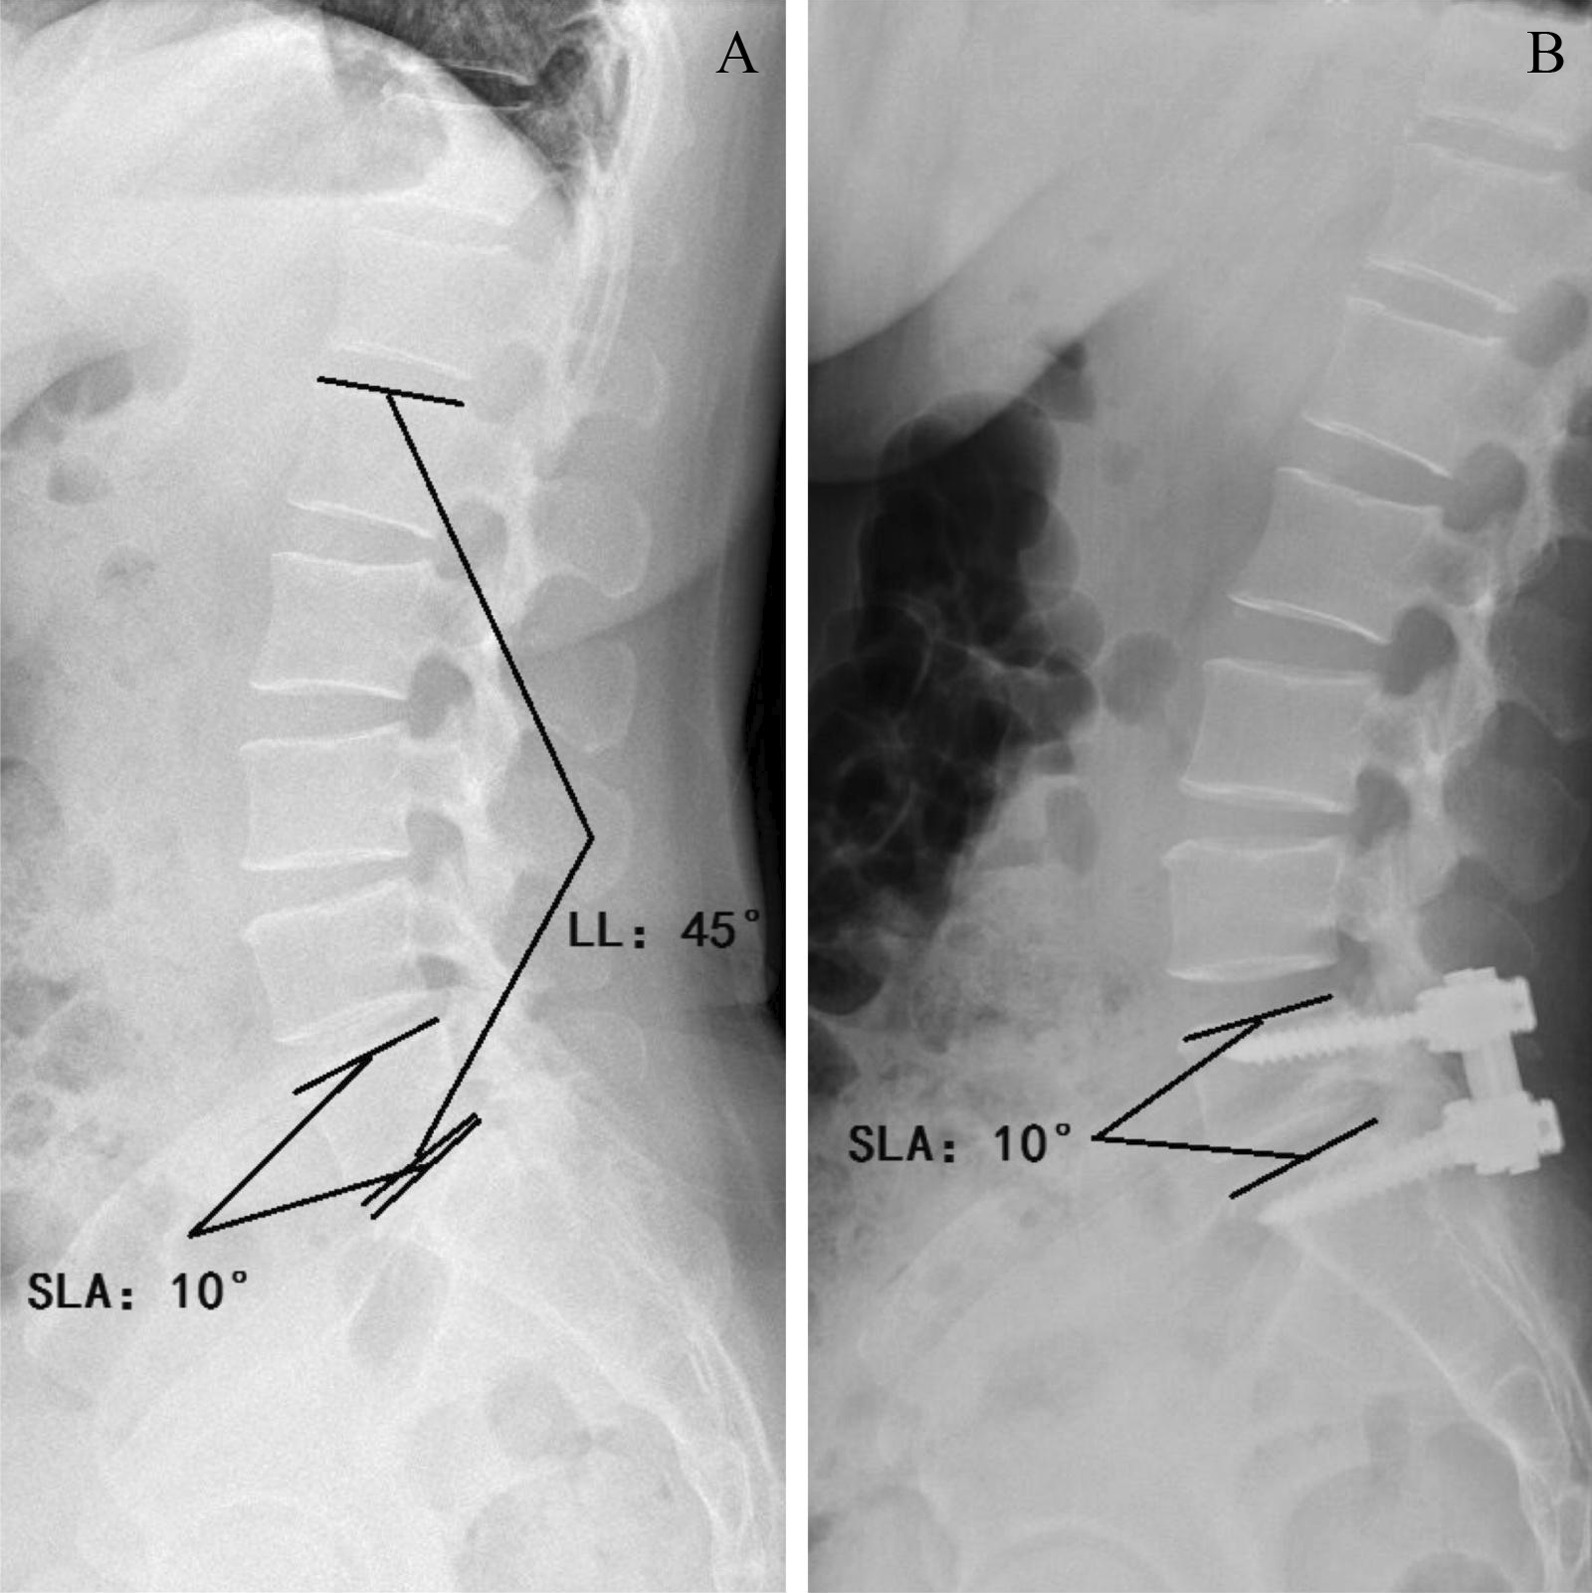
Preoperative and immediate postoperative lateral lumbar radiograph. A Preoperative lateral lumbar radiograph. The method of measuring SLA and LL is marked in picture. B Immediate postoperative lateral lumbar radiograph
